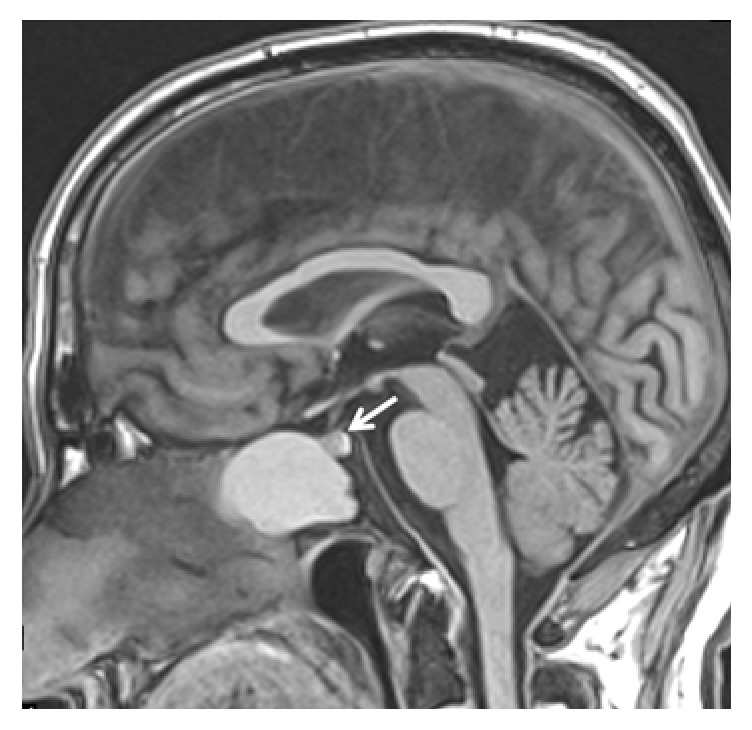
T1-weighted RMI in sagittal cut showing expansive cystic mass occupying the sphenoid sinus and posterior ethmoid. Observe the integrity of pituitary gland (white arrow).
